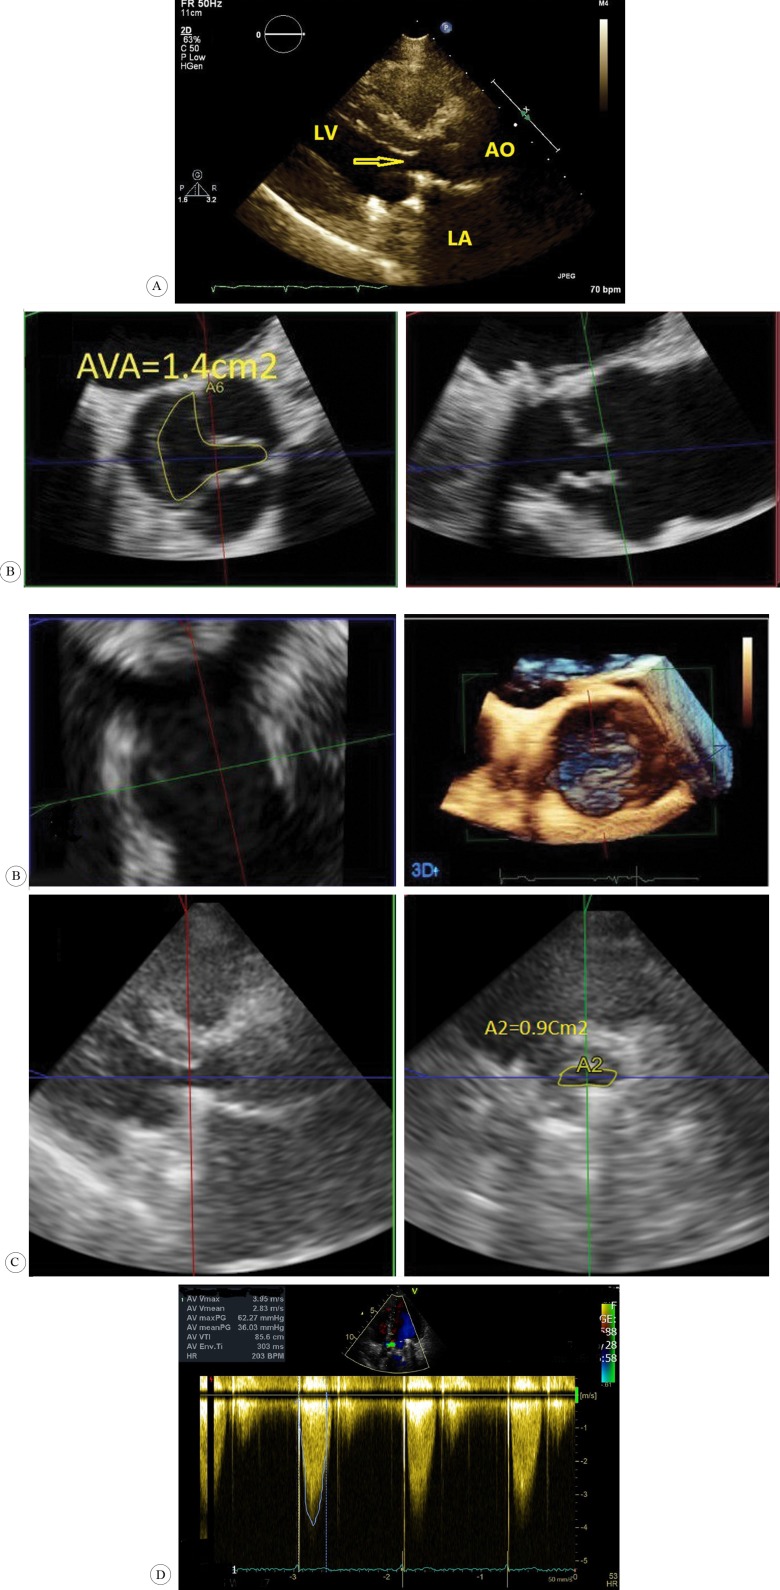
A) Anterior displacement of the mechanical prosthetic mitral valve, resulting in the left ventricular outflow tract (LVOT) narrowing (arrow) in the parasternal long-axis view in transthoracic echocardiography; B) Direct planimetry of the aortic valve in three-dimensional (3D) transesophageal echocardiography in the mid-esophageal short-axis of the aortic valve view; C) Direct planimetry of the LVOT using 3D transthoracic echocardiography in the parasternal long-axis view; and D) The LVOT pressure gradient in the 5-chamber view in transthoracic echocardiography evaluated by continuous wave
